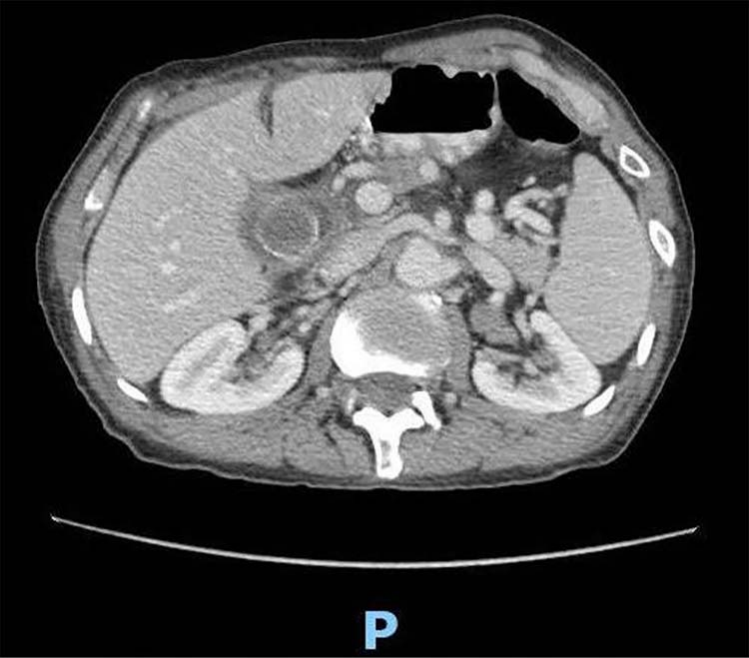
Enhanced CT scan of the abdomen confirming the presence of a 2.5-cm gallstone.
